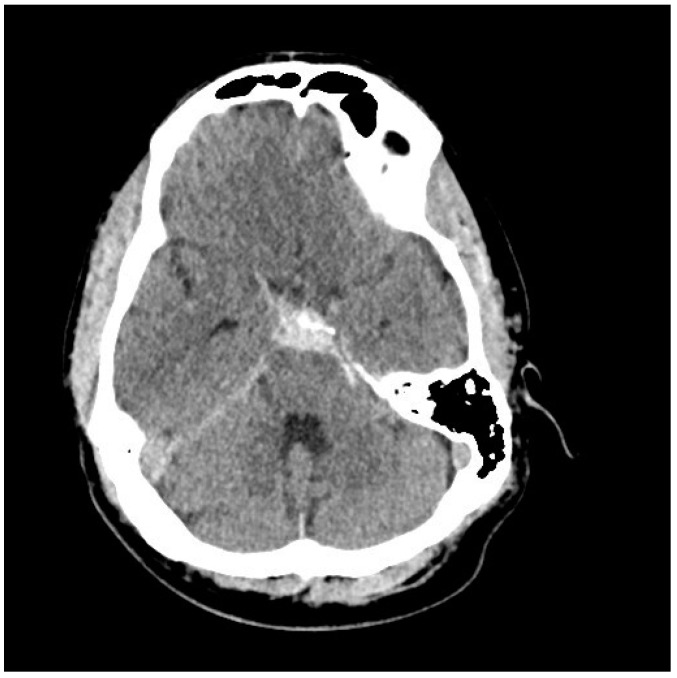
CT scan showing focal hyperdense subarachnoid clots near the midbrain and tentorial incisura, typical of a peri mesencephalic SAH. The 51-year-old patient presented with a GCS of 15 following a thunderclap headache. CT angiography was normal.
